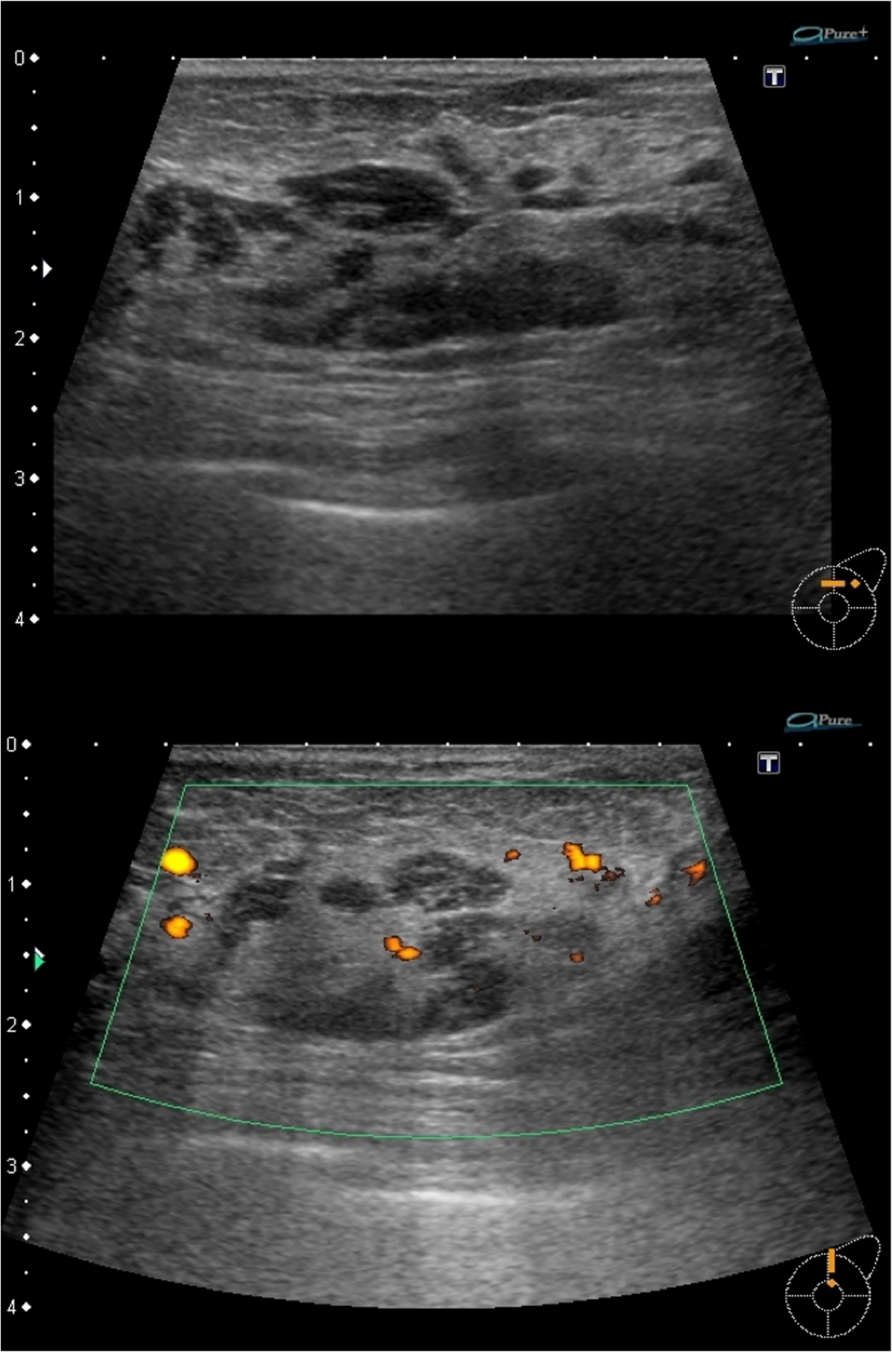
Ultrasonography of the left breast. The C region of the left breast contained an irregularly shaped, poorly echoic region. Large- and small-sized irregularities were observed over a wide range that excluded the B region. The mammary gland tissues clearly differed in both properties and thickness between the left and right sides. A poor blood flow signal was observed on Doppler ultrasonography. Swelling and thickness were observed in several axillary lymph nodes; however, the cortical echo level was low and internal heterogeneity was observed mostly using the lymph node gate; this finding was not one-sided
